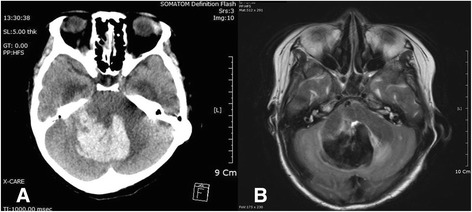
Brain computed tomography (a) and magnetic resonance imaging (b) revealing acute hemorrhage with perilesional edema in the bilateral cerebellum causing obstructive hydrocephalus, suggestive of mycotic aneurysmal rupture
